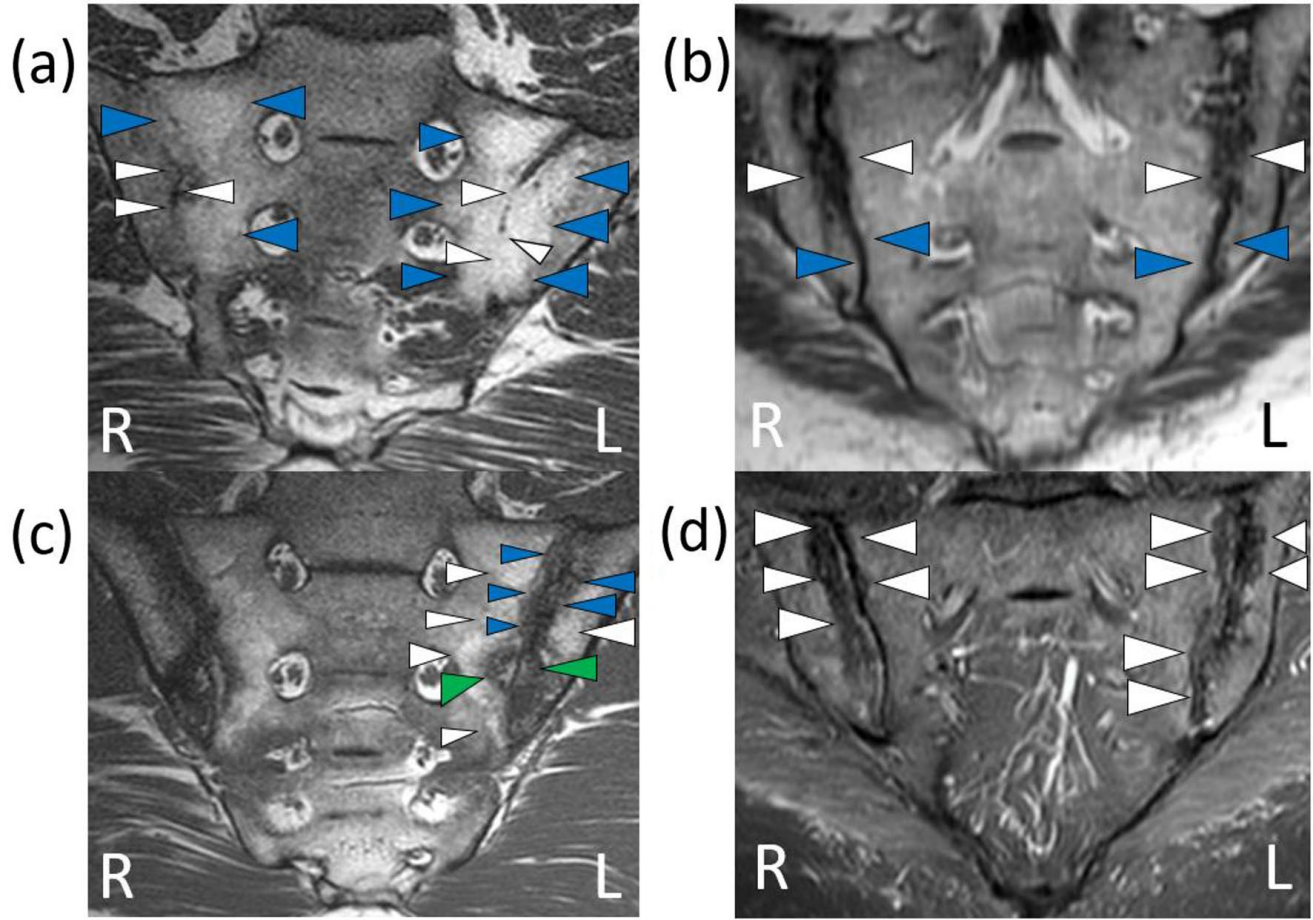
Findings compatible with chronic sacroiliitis on MRI of the sacroiliac joints. Source: the authors (2019). Coronal T1 sequence: bilateral reduction of the intra-articular space in the sacroiliac joints consistent with bone bridges (white arrows), and bilateral high signal intensity suggestive of fat metaplasia (blue arrows) (a). Coronal T1 sequence: low signal intensity bilaterally consistent with bone erosion (white arrows) and bone sclerosis (blue arrows) (b). Coronal T1 sequence: on the left high signal intensity consistent with fat metaplasia (white arrows) and low signal intensity suggestive of bone erosion (green arrows) and bone sclerosis (blue arrows); similar findings on the right, with lower signal intensity (c). Coronal STIR sequence: low signal intensity bilaterally consistent with bone erosion (white arrows) (d). R: right side , L: left side.
